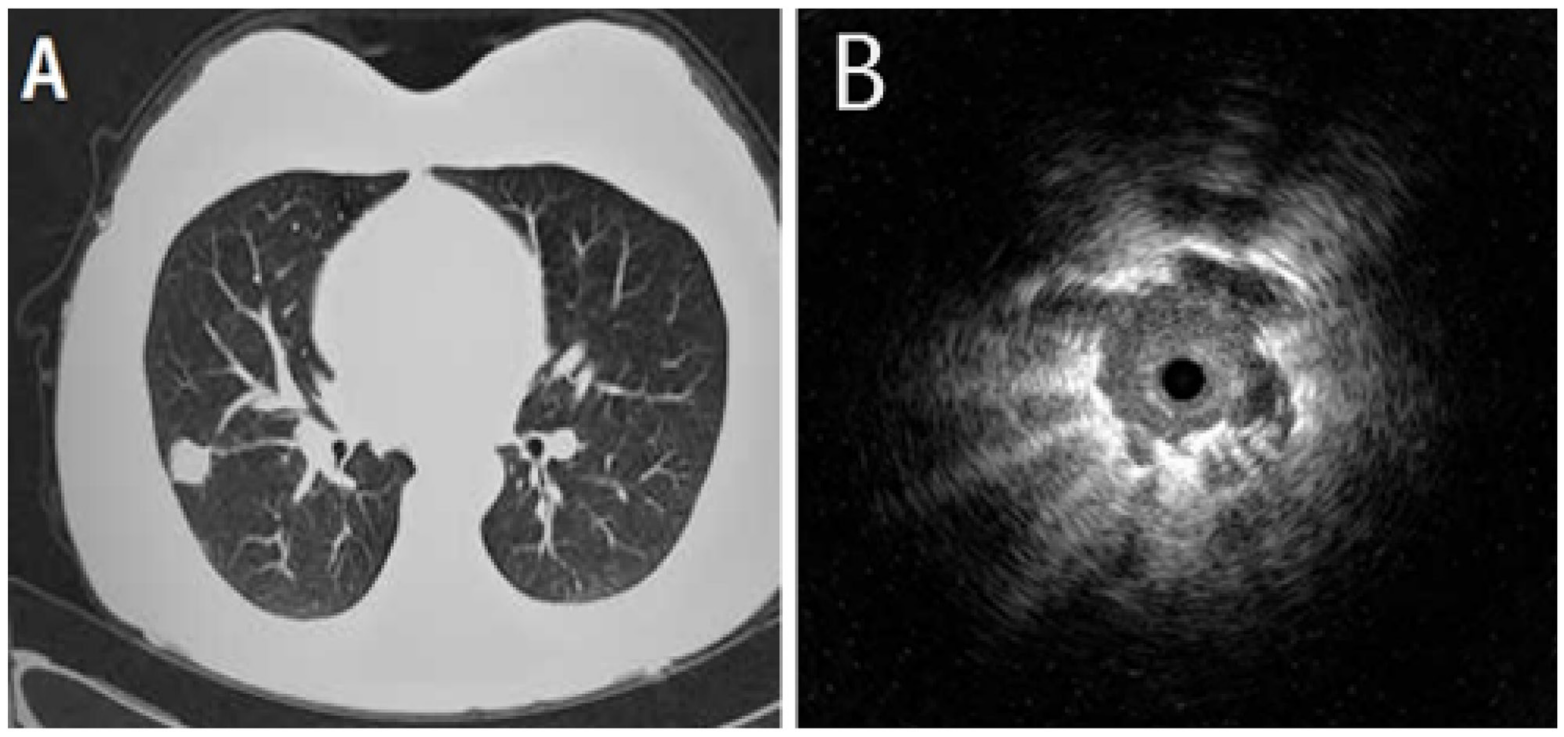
A 52-year-old male who underwent right middle lung lobectomy for pulmonary adenocarcinoma (A) Chest computed tomography showed a pulmonary nodule of 26 mm in diameter. (B) Endobronchial ultrasonography showed a low echoic nodule surrounded by a strong reflected interface produced between the aerated lung and the lesion.
